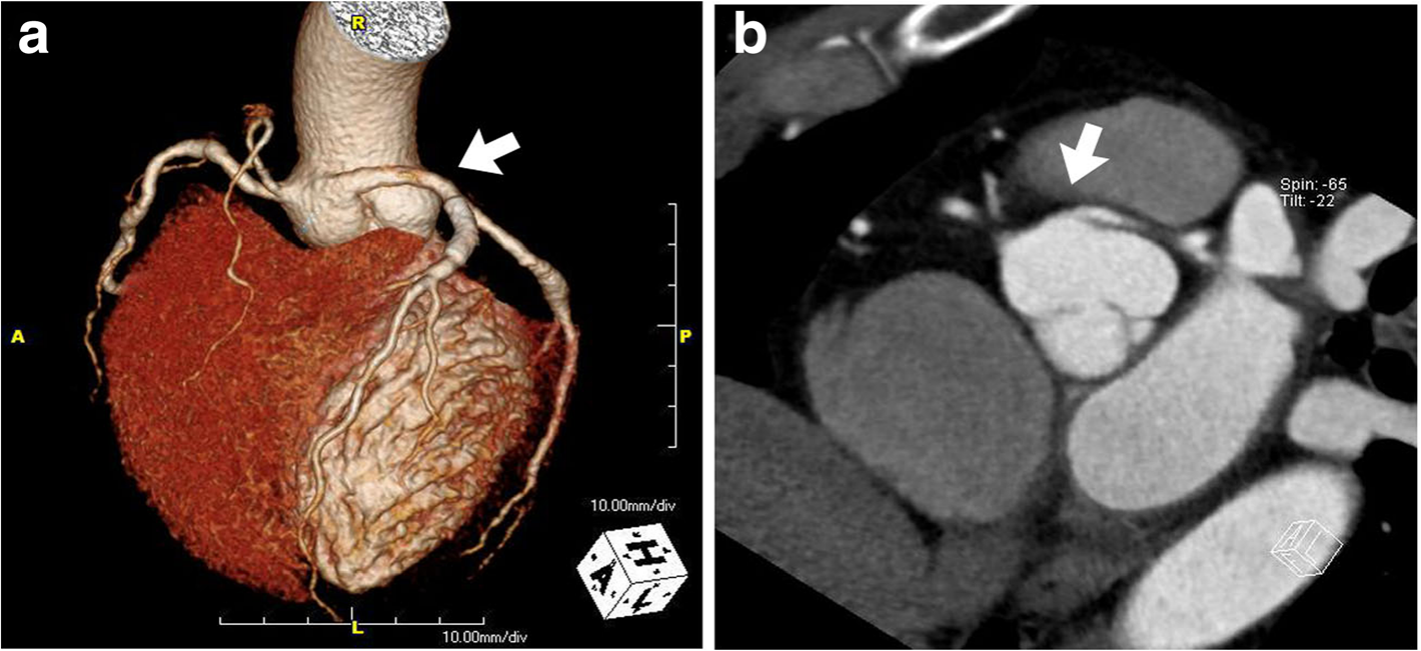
a Computed tomography coronary angiography revealed anomalous origin of the left coronary arteries from the right coronary cusp (*arrow*) in three-dimensional reconstructed image. b Computed tomography coronary angiography showed the anomalous origin of left coronary artery and traveling behind the pulmonary artery (*arrow*)
